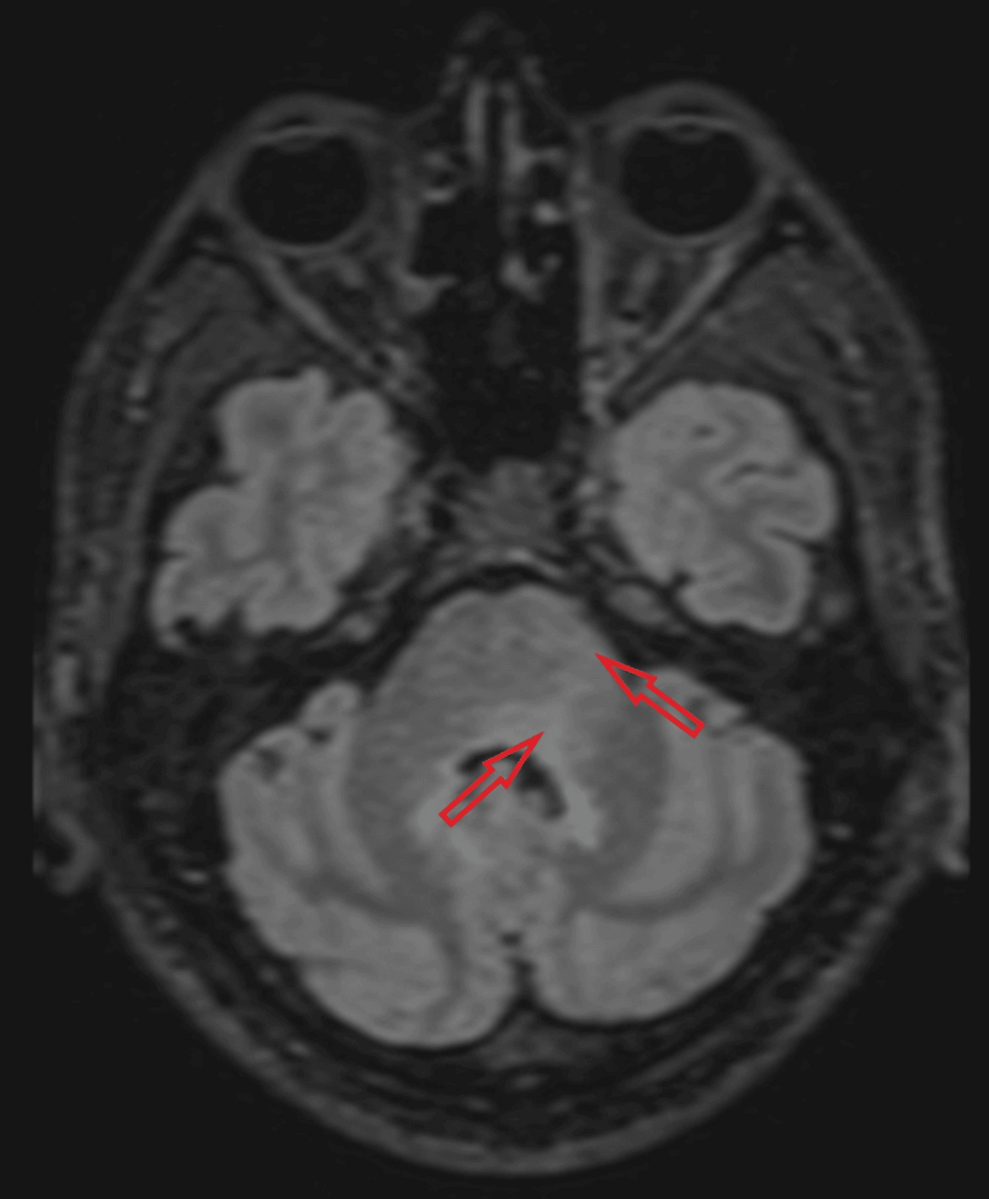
Axial fluid-attenuated inversion recovery (FLAIR) MRI showing patchy signal hyperintensity and edema around the fourth ventricle (left greater than right) and left cerebellar peduncle.
